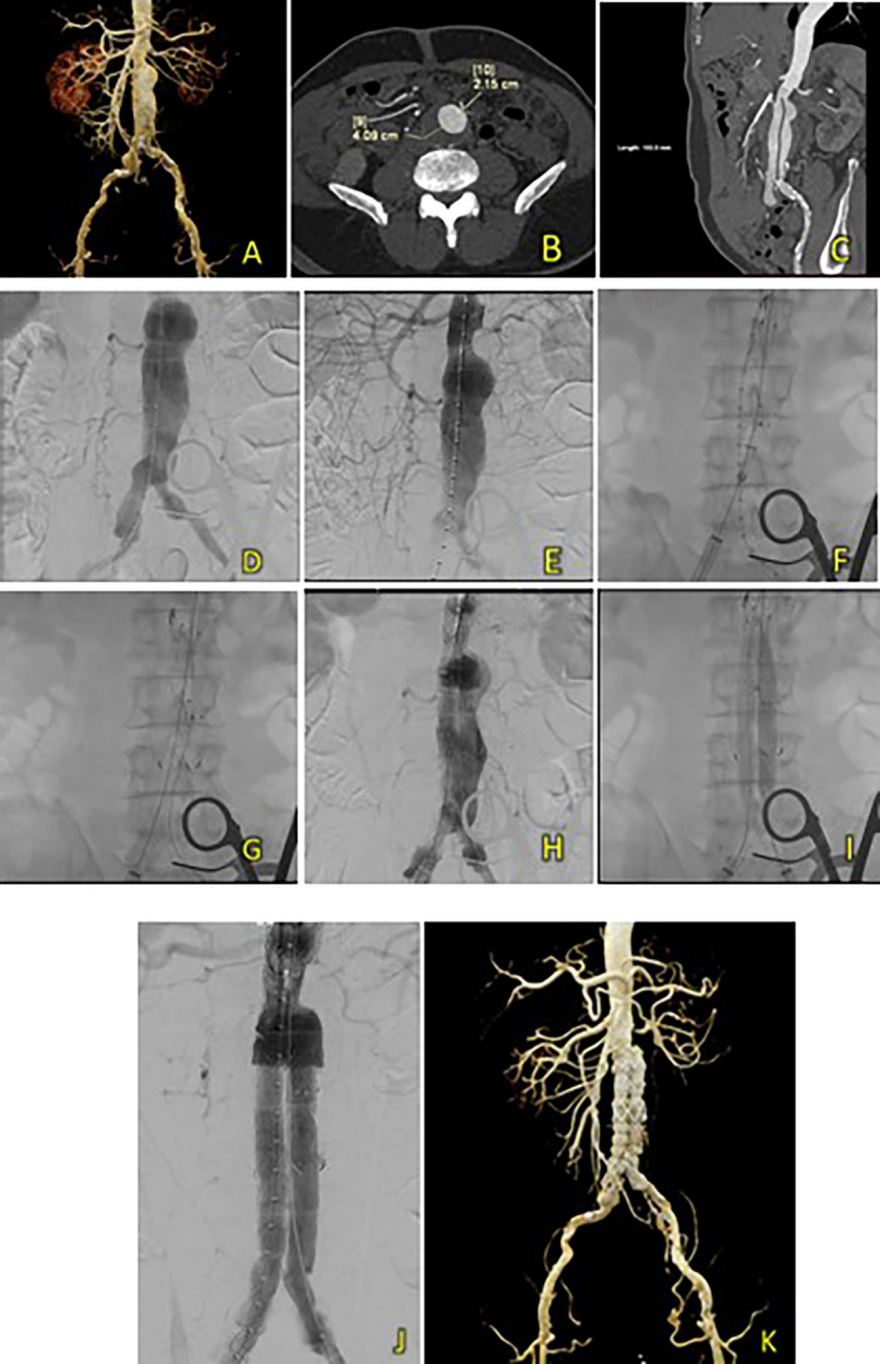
T&K B-EVAR on Patient 4. (A) Pre-procedural CT scan. (B) Axial CTA. (C) Sagittal CTA. (D-I) T&K B-EVAR step-by-step. (J) Post-procedure aortography with good stent apposition and no endoleaks. (K) Post-procedural CT scan evaluation had good results with no signs of endoleaks.
